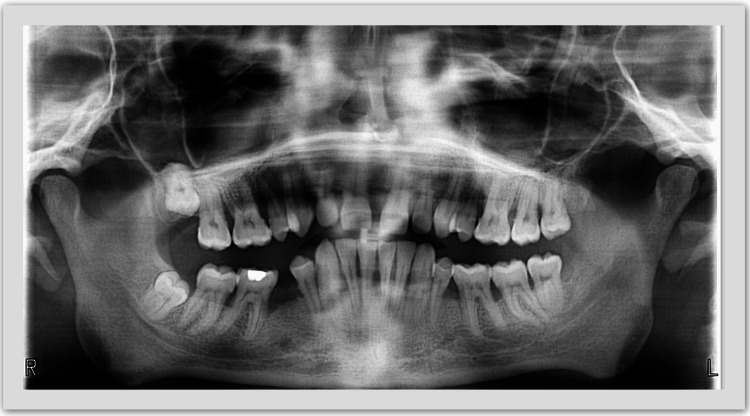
Patient's initial orthopantomography.
